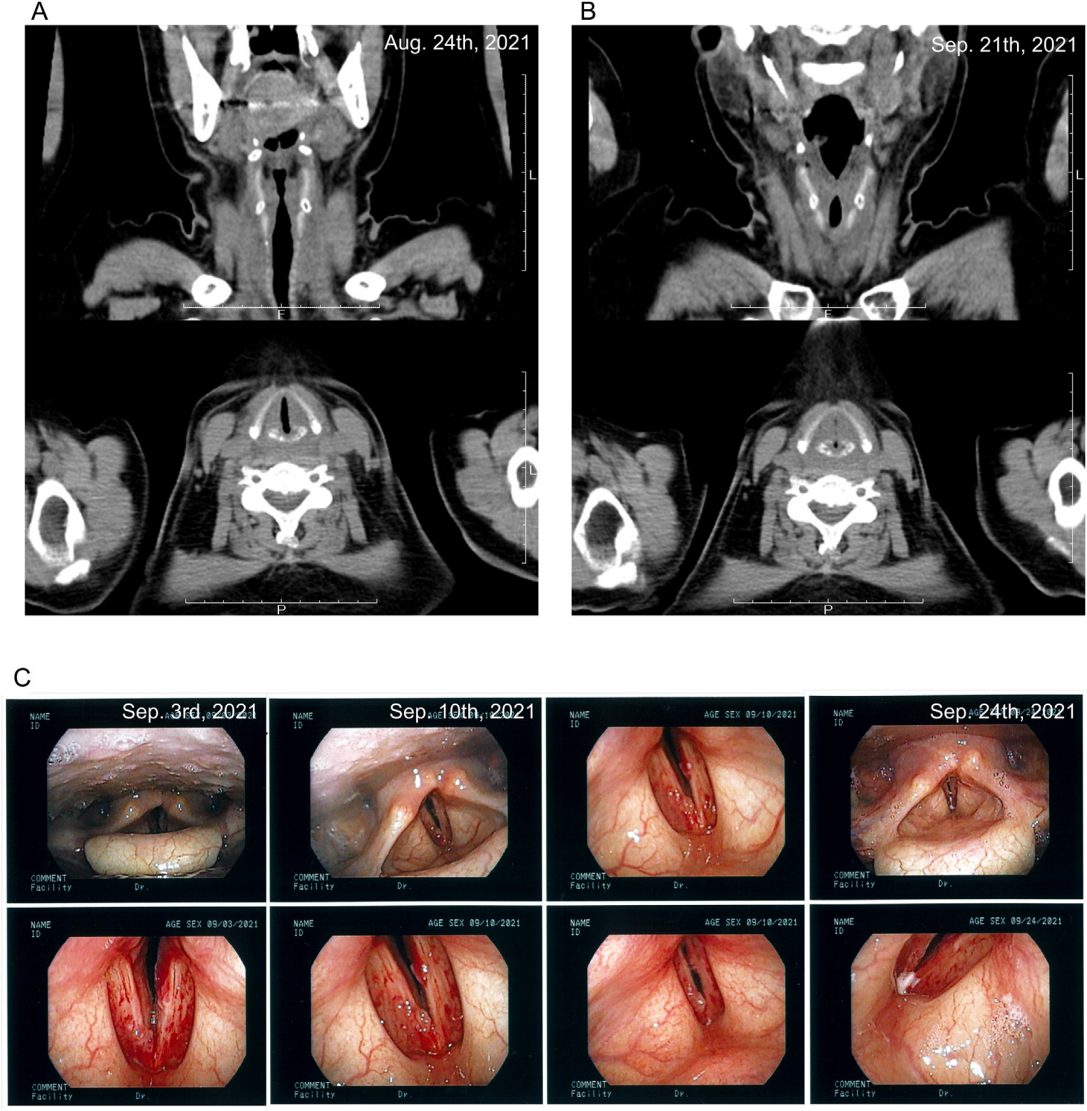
Computed tomography imaging and nasopharyngoscopy findings after COVID-2019 vaccination, showing computed tomography images at A, 5 days after COVID-19 vaccination and B, 2 weeks after steroid pulse therapy, as well as C, nasopharyngoscopy images before and after steroid pulse therapy.
